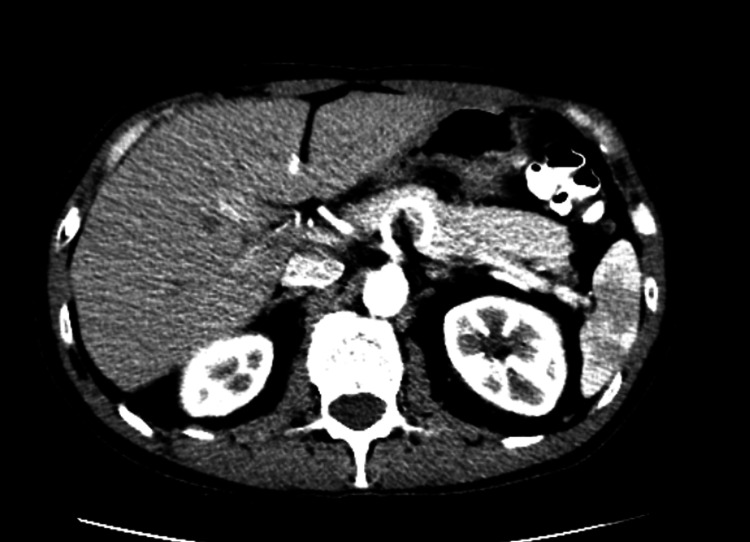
Surveillance CT abdomen pelvis showing resolution of liver metastasis, 07/12/2022
